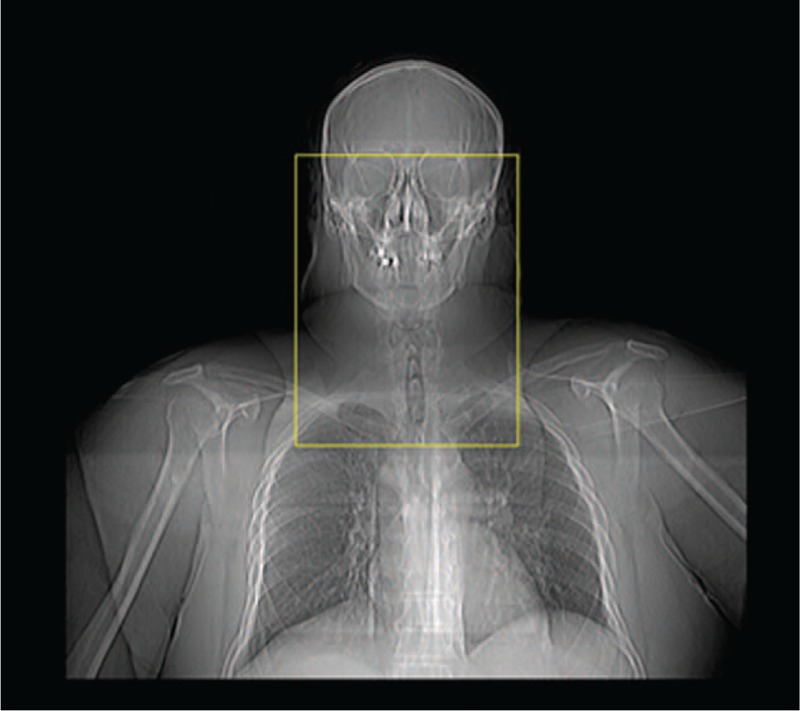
Computed tomography (CT) margins obtained from the scout view: from the roof of the bony orbits cranially, to the sternal angle of lewis inferiorly, and from the mid-clavicular interspace laterally.
